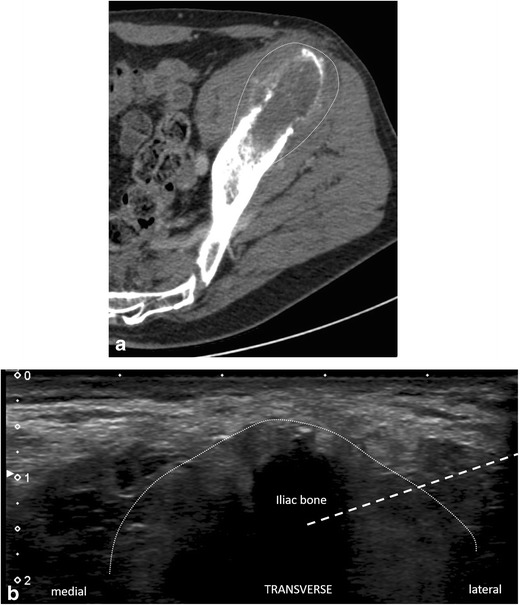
A 75-year-old man with clinical background of rectum adenocarcinoma presenting with a new lytic lesion in the iliac bone. a CT scan and b ultrasound show the osteolytic lesion with soft tissue extension (*dotted line*). An ultrasound-guided biopsy was made with a coaxial 14-G cutting needle (chosen path marked with a *dashed line*) and confirmed adenocarcinoma metastasis
